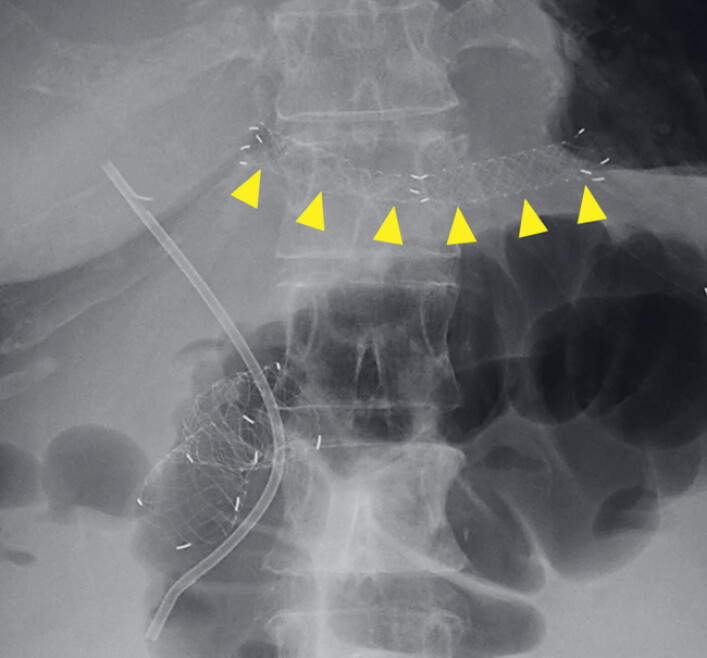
An abdominal X-ray 5 months after the MHSEMS placement showing no stent migration. MHSEMS, multihole self-expandable metal stent.
